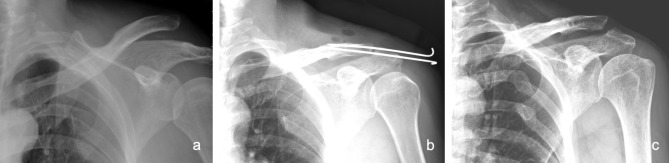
A 44 years old male presented with left shoulder residual subluxation after 4 months of K-wires removal. (a) Preoperative plain radiograph. (b) Status post open reduction and fixation with K-wires and CC ligament augmentation with Mersilene tape. (c) Residual subluxation after 4 months of K-wires removal. However, there was no clinical instability of the patient shoulder
